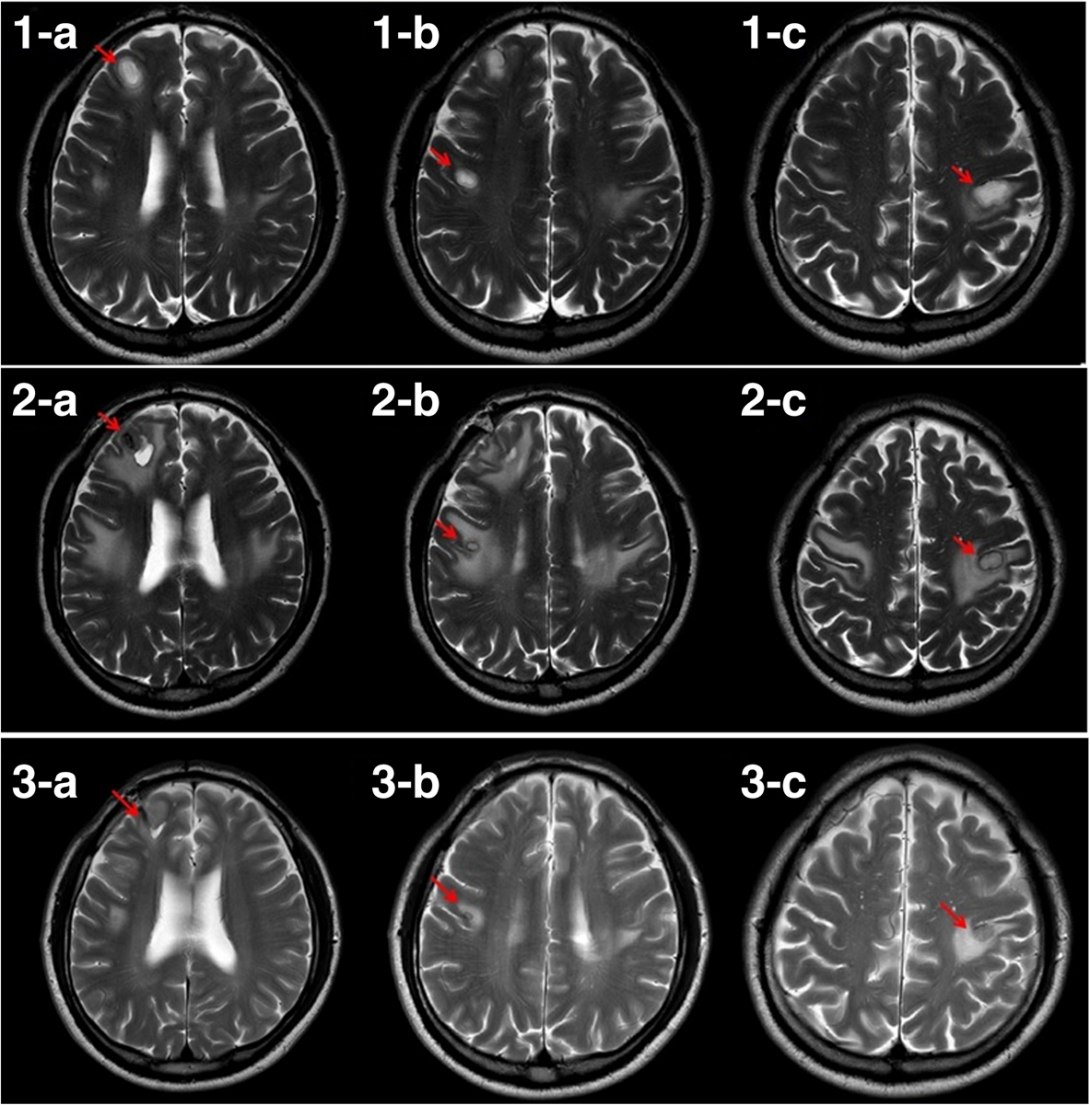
Magnetic resonance images of a 45-year-old male admitted with right facial spasm, tingling and twisting of the right arm, paresthesia, and dysarthria. A high T2-weighted signal was observed in the right mid-frontal region and in the left and right high-frontal subcortical white matter (WM). Top: three regions were well-defined, approximately 13 mm, 9 mm, and 15 mm low-density ovoid lesions, with diffusion restriction and perilesional edema. Middle: the post-operative state; abscess drainage of the right mid-frontal lobe area. The size of several ovoid lesions decreased in both the left and right high-frontal subcortical WM. Bottom: after extraction of the periodontally involved teeth, the size of the lesions in the right mid-frontal and both the left and right high-frontal subcortical WM decreased significantly. A, right mid-frontal; B, right high-frontal; C, left high-frontal subcortical area. Red arrows identify the three brain abscesses
